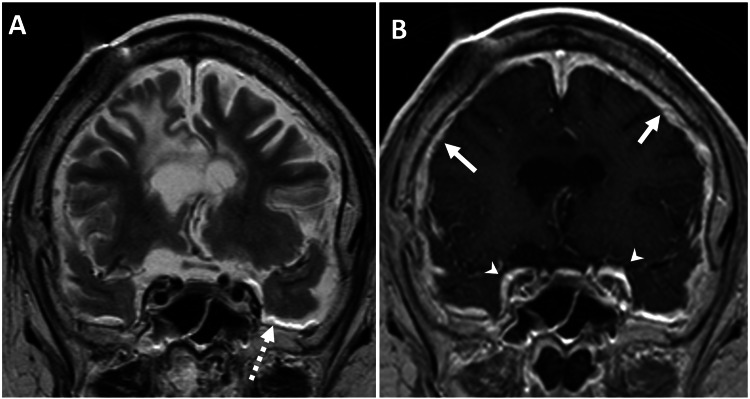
Coronal MRI demonstrating dural fat-fluid interface artifact and diffuse pachymeningeal enhancement in a patient with chronic VP shunting. An MRI of the brain was performed in a 66-year-old male with a medical history of altered mental status, in the context of known severe mental retardation, cerebral palsy, and a VP shunt. (A) Coronal T2-weighted MRI brain images show barely appreciable dural T2 hyperintensity along the falx, which is separable from the T2 hyperintense CSF signal. However, there is a chemical shift artifact related to the fat-CSF fluid interface along the bilateral middle cranial fossa (dashed white arrow). (B) Coronal T1-weighted contrast-enhanced MRI brain image, after intravenous injection of 20 mL of gadoteridol (ProHance; Bracco, Milan, Italy), shows diffuse pachymeningeal dural enhancement along the middle cranial fossa (white arrowheads) and cerebral convexities (solid white arrows). Additionally, there is diffuse atrophy of the brain parenchyma, with prominent bilateral lateral ventricles related to known cerebral palsy. A susceptibility artifact in the right frontal scalp is related to the VP shunt catheter reservoir. MRI, magnetic resonance imaging; VP, ventriculoperitoneal; CSF, cerebrospinal fluid
